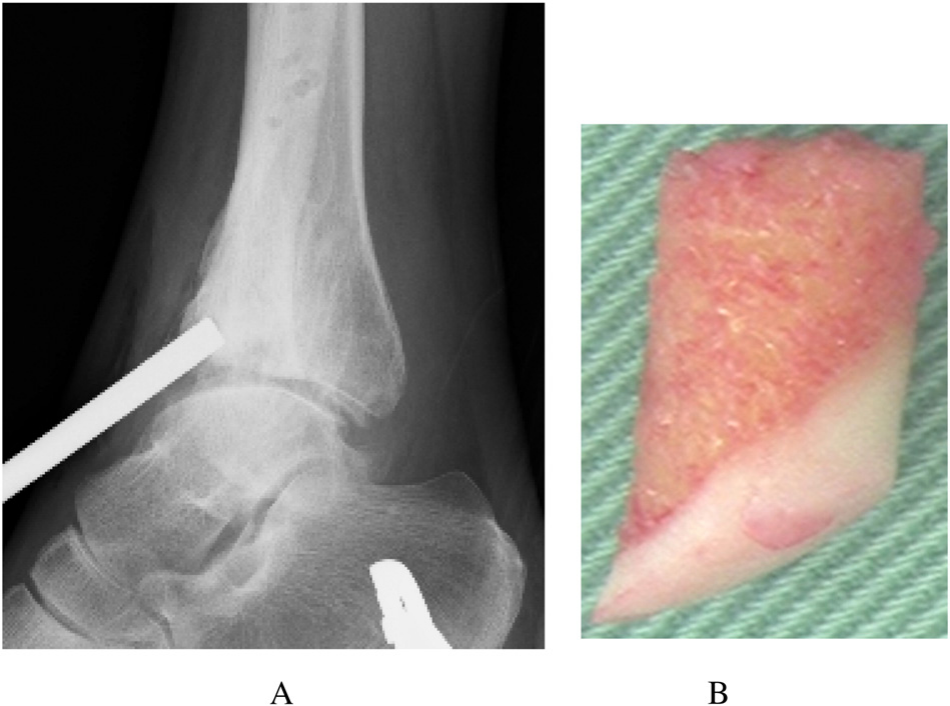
Based on a radiograph taken to calculate the inclination angle of the dilator, two 8-mm osteochondral autograft plugs ramped 35° and 45° were harvested: (A) radiograph at surgery, (B) a harvested plug ramped 35°.
